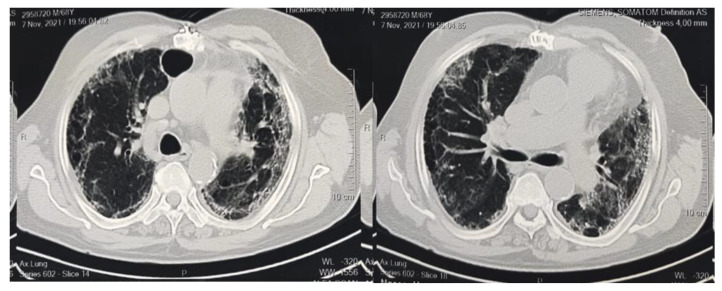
CT imaging indicates bilateral marked pulmonary infection with diffuse ground glass opacities, areas of air trapping, and pulmonary thickened interstitium with subsequent architectural distortion, basal bronchiectasis, and honeycombing appearance.
